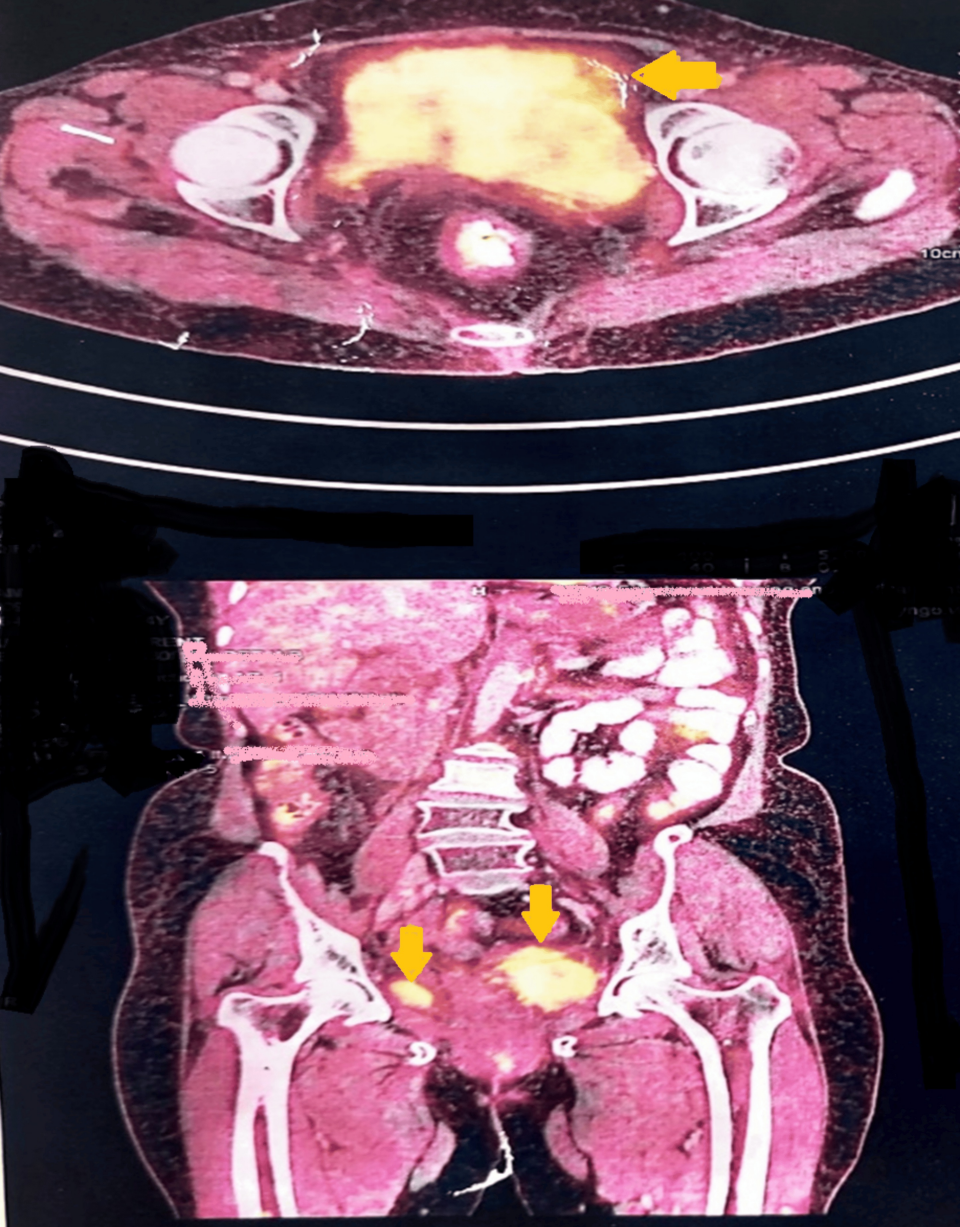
PET/CT image showing increased FDG uptake by the tumor (Case 4). PET/CT: positron emission tomography/computed tomography; FDG: fludeoxyglucose-18.
